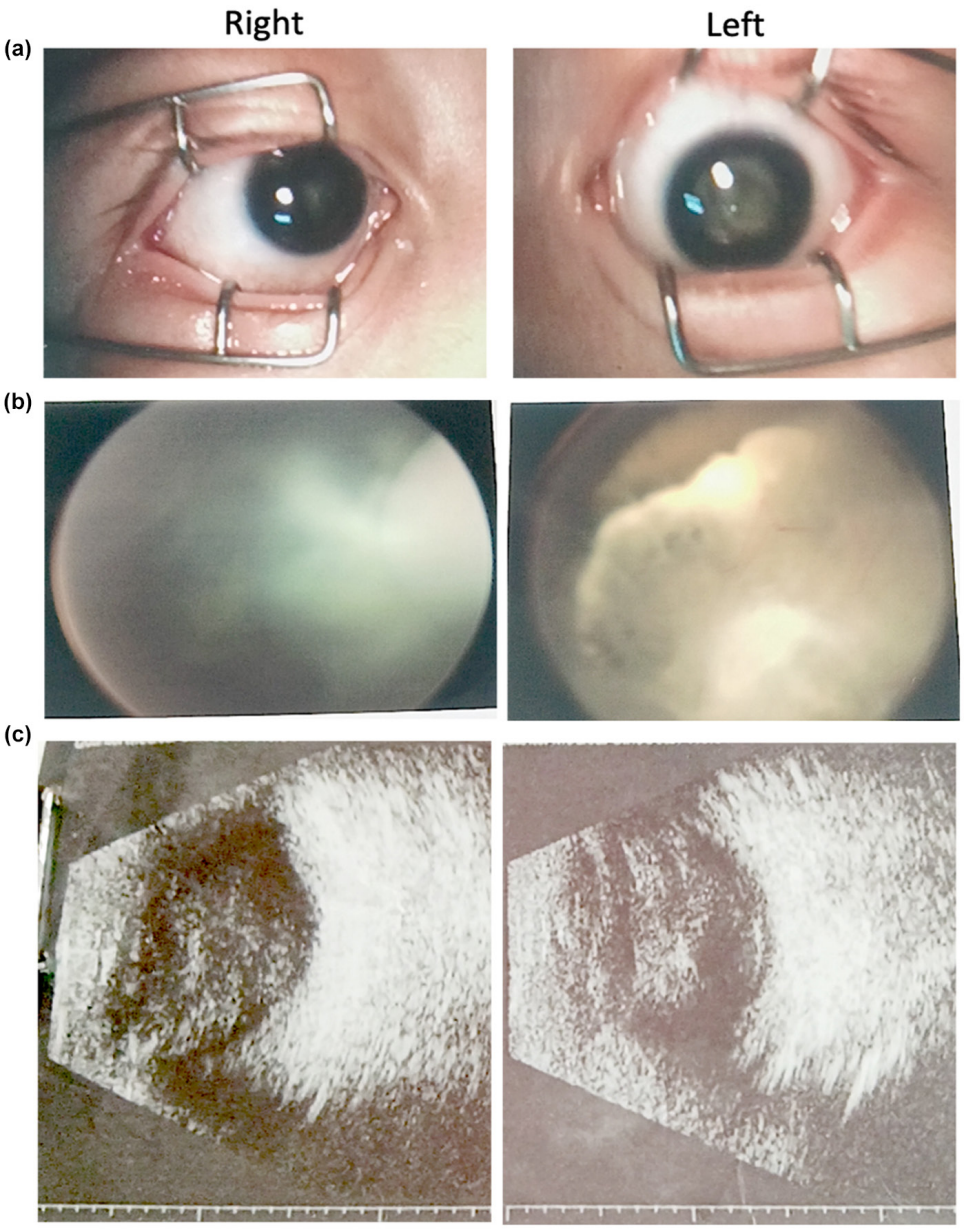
Eye examination in the patient. (a) Iris coloboma. (b) Ophthalmoscope shows the choroidal coloboma. (c) Ophthalmic ultrasound showed vitreous opacity in both eyes and left retinal detachment
